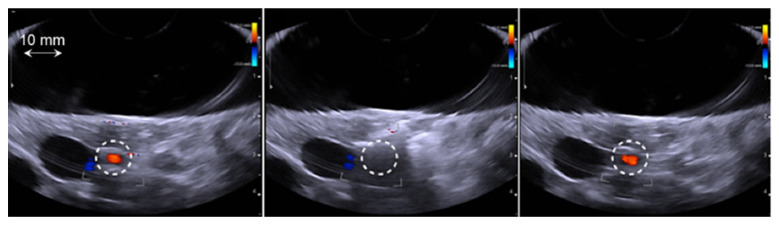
Typical example in group 4 of a temporary spasm. From left to right: Color Doppler imaging of the targeted artery (white circle) just before the HIFU treatment, just after the HIFU sonication and 12 min after the sonication. Normal blood flow was recovered on average 12 min after the temporary spasm in all cases.
